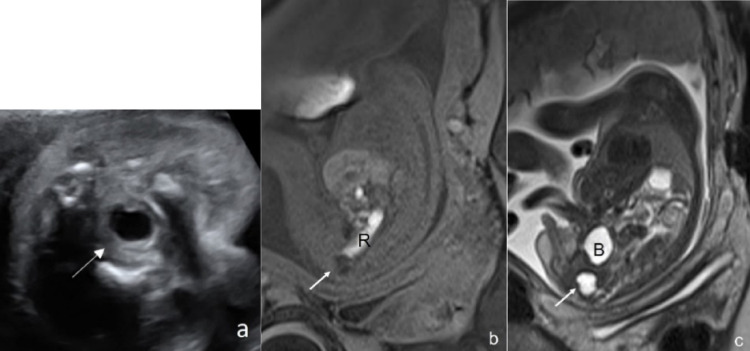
Imaging findings in rectal duplication cyst. Axial US image (a) at 25GW shows a pre-sacral located, well-defined cystic lesion in the fetal pelvis (arrow), with a thick wall, but provides limited value in evaluation of the adjacent organs. Midline sagittal T1- (b) and T2-WI (c) images of MRI at 30GW confirm the thick wall of the cyst (arrows) and show its direct anatomical relationship with the rectum (R), filled with normal meconium, (B: Bladder). The absence of contact with the coccyx excludes sacrococcygeal teratoma
